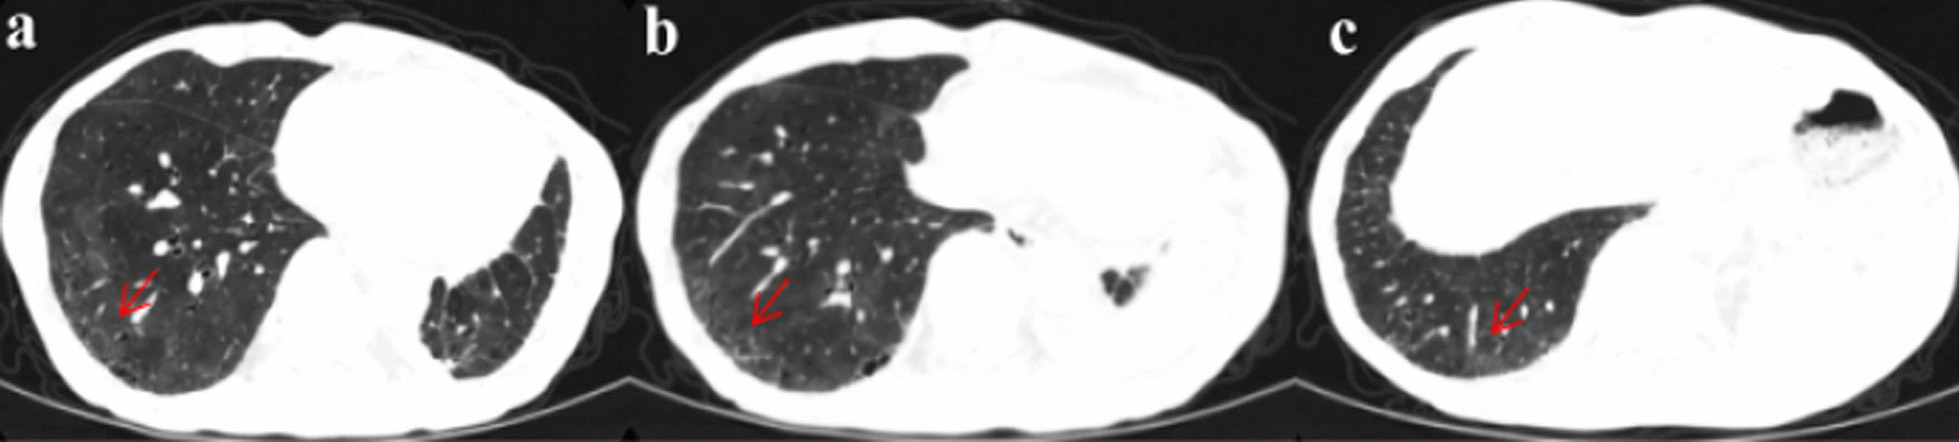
a–c Chest CT showed interstitial changes in the lower lobe of the right lung after oral administration of almonertinib for 3 months
